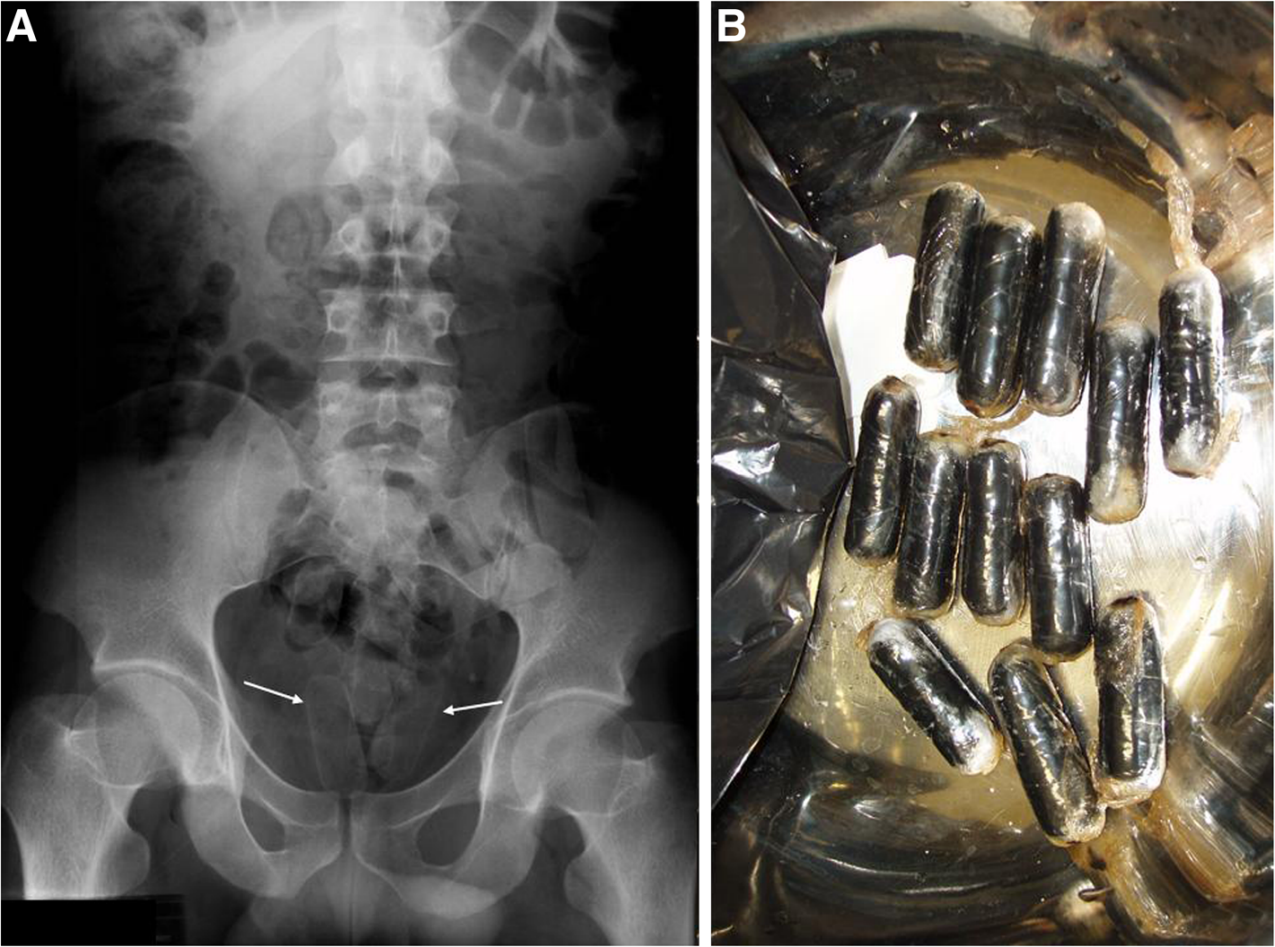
Cocaine packages hidden in the patient intestine. A- Plain abdominal radiograph performed on the day of patient admission showing several foreign bodies located in the sigmoid colon (white arrows); B- Small cocaine packages evacuated by the patient.
